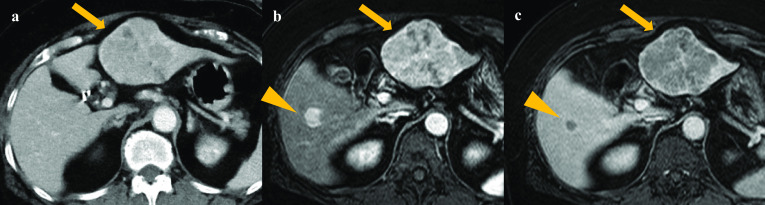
Preoperative imaging of the liver tumors. The enhanced CT scan showed an 81 × 63-mm-sized tumor in segment 3 (a) (arrow). The rim of the tumor was enhanced, and the inside of nodules showed hypoattenuation. Another 17 × 12-mm-sized tumor was found in segment 5 (b, c) (triangle) in addition to the liver in segment 3 (arrow) on Gd-EOB-DTPA-MRI. Both tumors were highly enhanced in the early arterial phase (b), followed by washout in the delayed phase (c). *CT* computed tomography; *Gd-EOB-DTPA* gadolinium ethoxybenzyl diethylenetriamine pentaacetic acid; *MRI* magnetic resonance imaging
